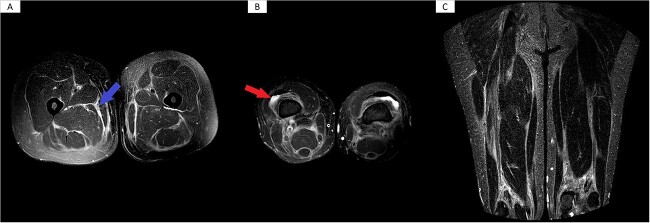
Bilateral MRI of the thighs. (A) MRI showed subcutaneous and deep interstitial edema, as well as mild muscular edema (Arrow on FIgure A). (B) There is evidence of mild bilateral knee joint effusions (Arrow on Figure B). (C) A few left thigh varicose veins are also noted.
